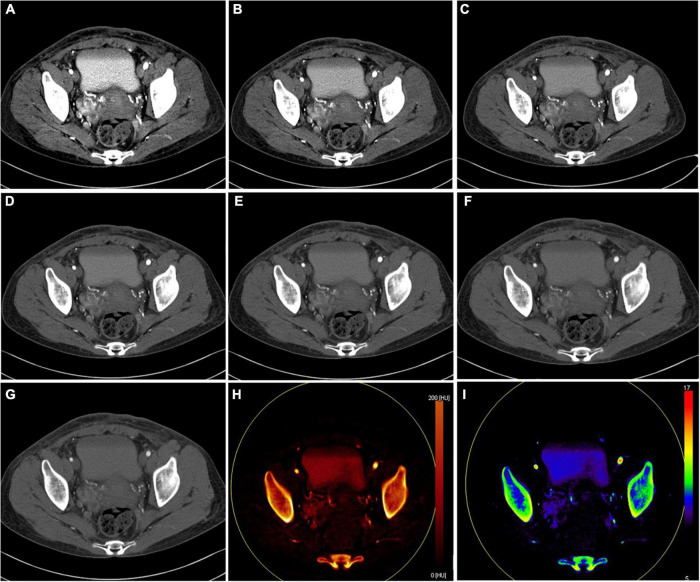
Axial dual-energy computed tomography (CT) images show tortuous and enlarged paracervical veins. (A–G) 40–100 keV VMIs (10-keV interval). (H) Iodine density map. (I) Z effective map.
